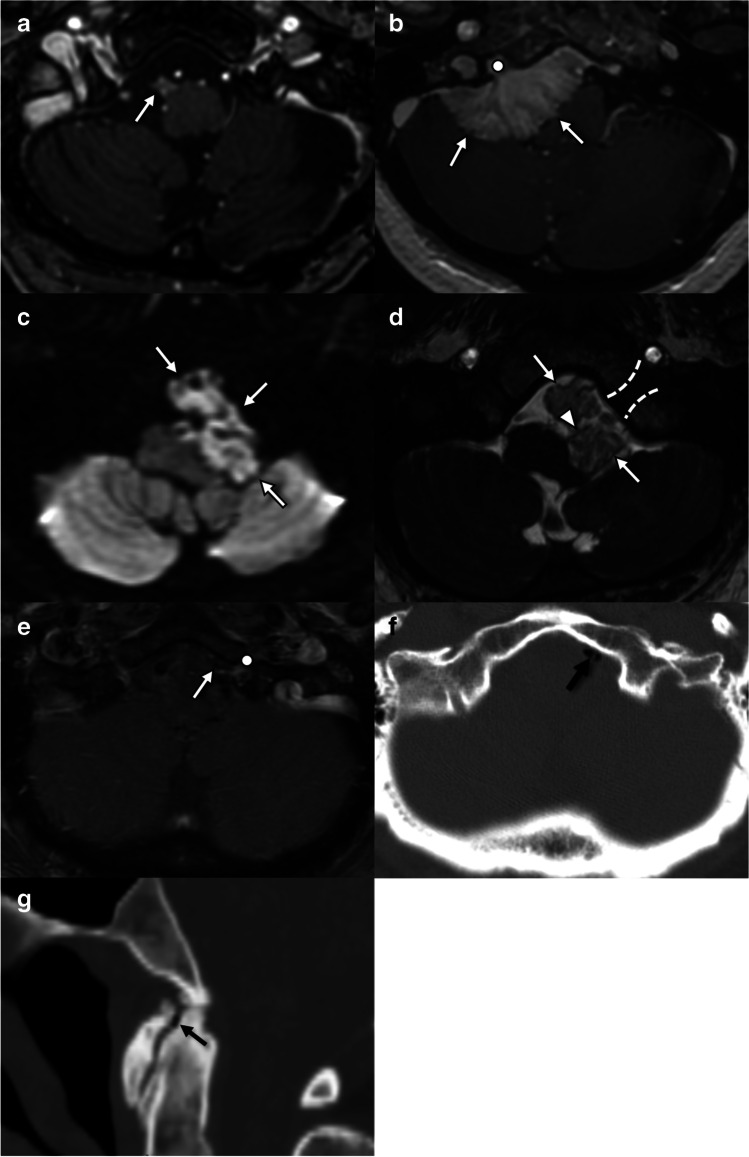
Lesions involving the cisternal segment of the hypoglossal nerve. a Axial post-gadolinium T1W SPGR sequence in a 44-year-old with history of NF2 and prior left vestibular schwannoma resection demonstrates an enhancing nodule arising from the right lateral medulla from a hypoglossal nerve root. b Axial post-gadolinium T1W SPGR sequence in a 66-year-old with multiple right-sided cranial nerve palsies demonstrates a homogenously enhancing lobulated dural-based mass (arrow) arising from the right petrous face and extending into the hypoglossal canal (dot). Surgical pathology confirmed WHO grade 1 meningioma. c Axial DWI (*b* = 1000) in a 14-year-old female with headache and slowly progressive lower cranial neuropathies demonstrates a lobulated extra-axial cystic lesion in the left cerebellomedullary cistern with markedly reduced diffusion (arrows) consistent with an epidermoid cyst. d Axial FIESTA in the same patient as c clearly shows left hypoglossal nerve rootlets (arrowhead) traversing the epidermoid cyst (arrows) en route to the hypoglossal canal (dashed lines). e Axial post-gadolinium T1W in a 54-year-old with chronic left-sided tongue weakness is notable for a non-enhancing T1 hypointense extradural lesion (arrow) at the orifice of the left hypoglossal canal (dot). This was of low signal intensity on T2W (not shown). f Axial unenhanced CT in the same patient as e reveals the lesion is of gas density (black arrow). g Sagittal reformatted unenhanced CT image of the midline skull base in the same patient as e demonstrates marked degenerative changes at the cranio-cervical junction with intra-articular gas (black arrow). This was thought to have migrated to the extradural space in f and resulted in cisternal impingement of the hypoglossal nerve
